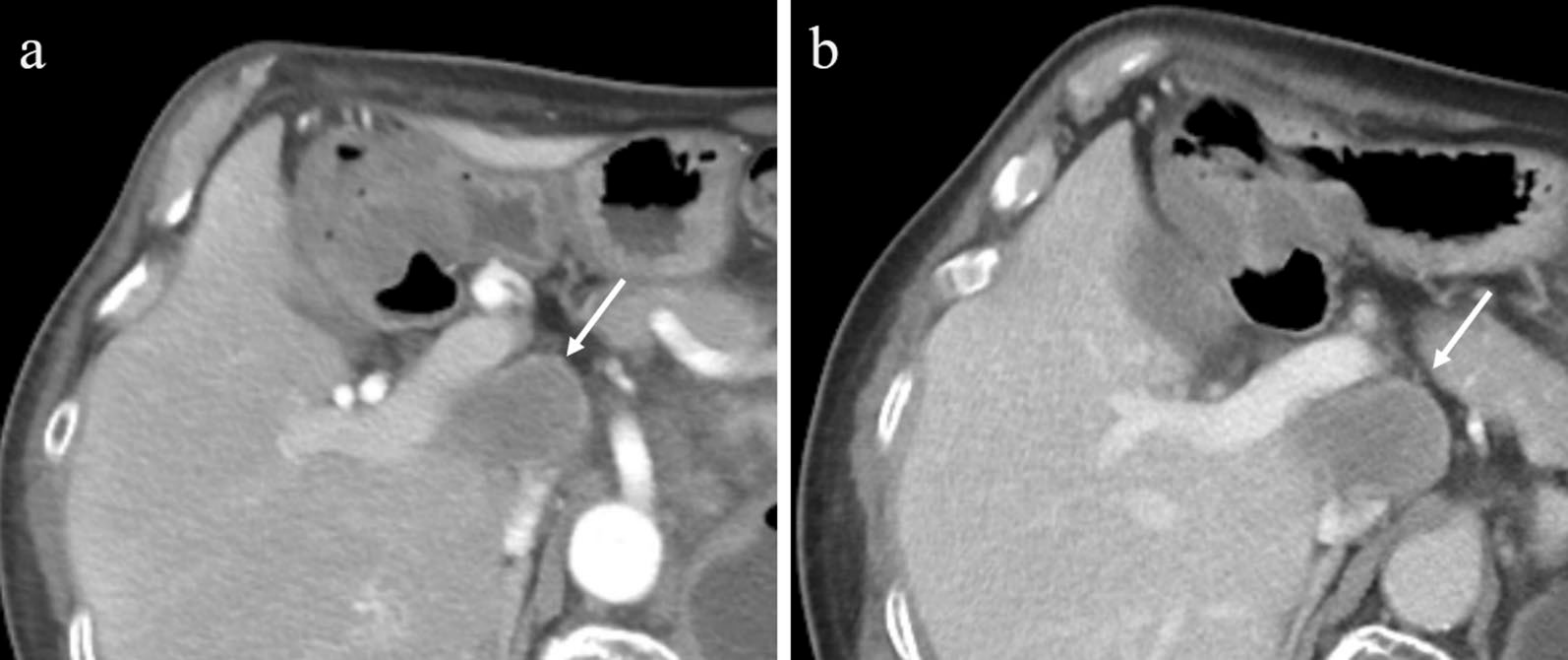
Abdominal contrast-enhanced computed tomography findings. a The tumor (white arrow) measuring 40 mm showed enhancement at the peripheral margin in the arterial phase. b The tumor (white arrow) with peripheral margin enhancement was prolonged in the delayed phase
